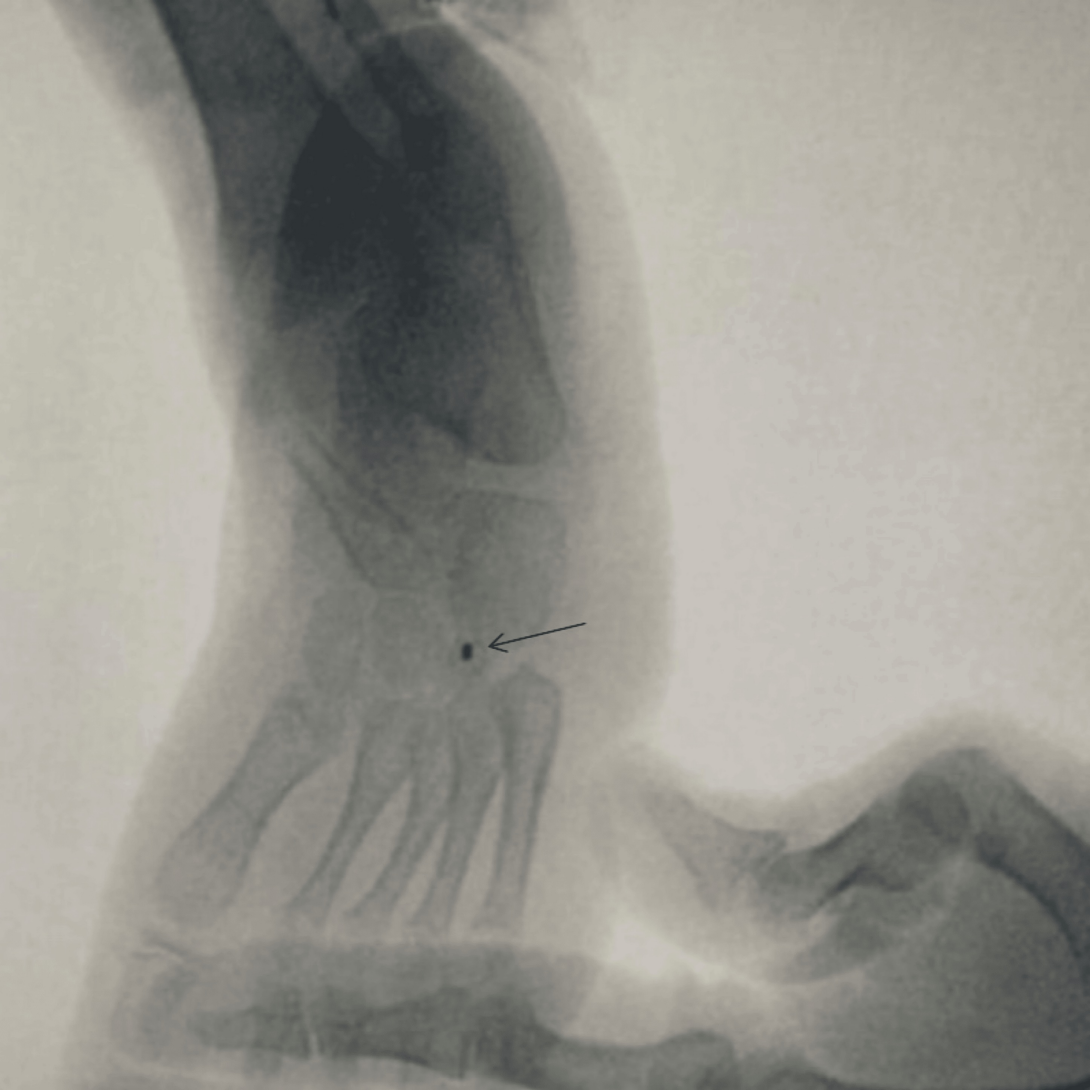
Intraoperative X-ray of the left foot during posterior tibial tendon transfer to the lateral cuneiform.
